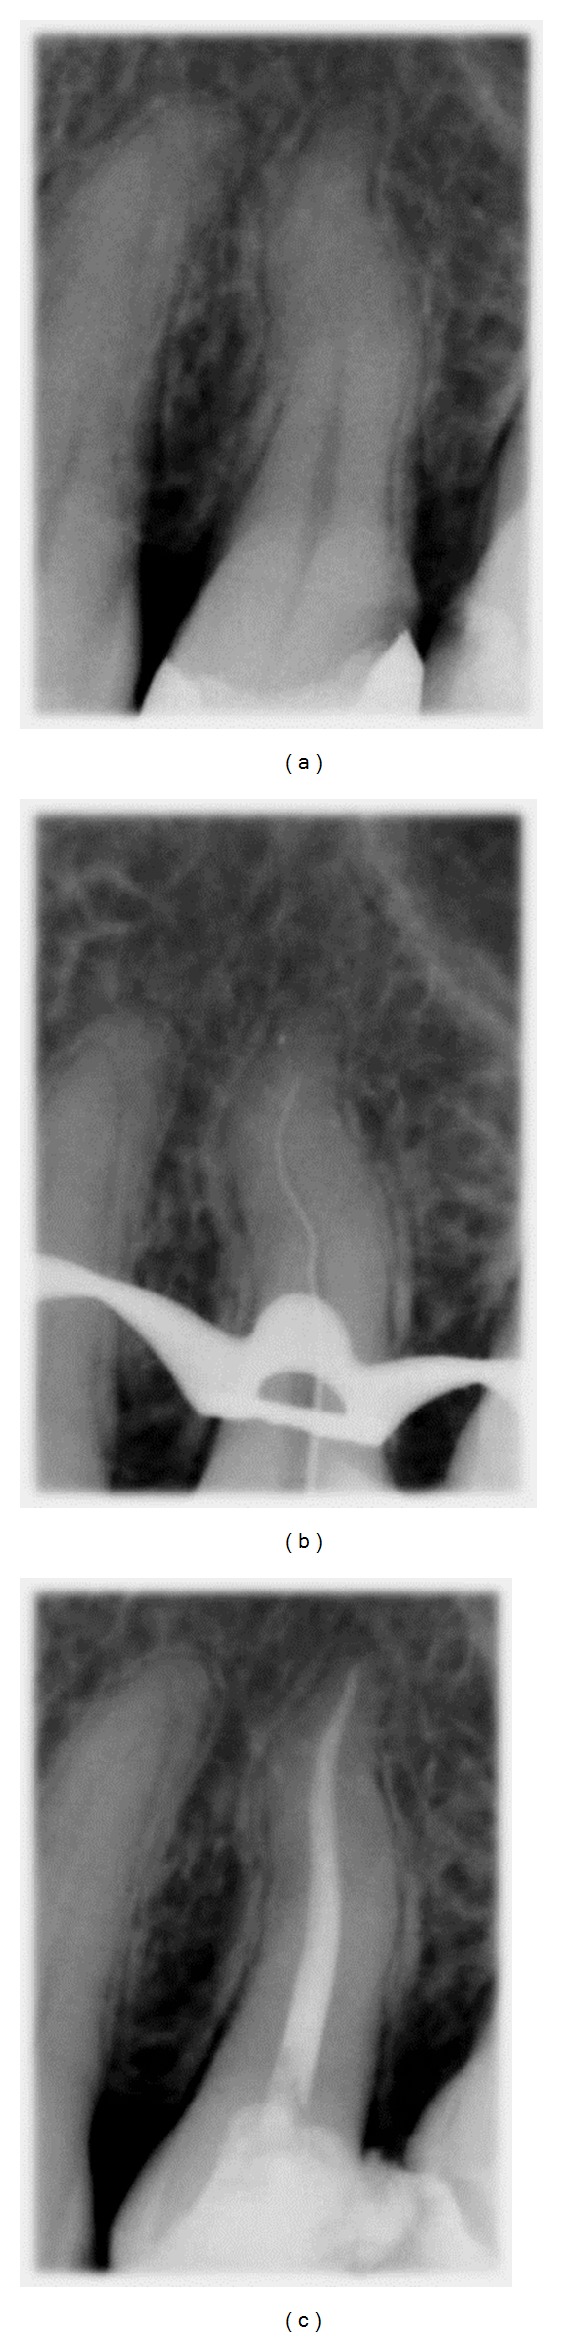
(a) Initial radiograph, (b) working length radiograph, and (c) final radiograph.
